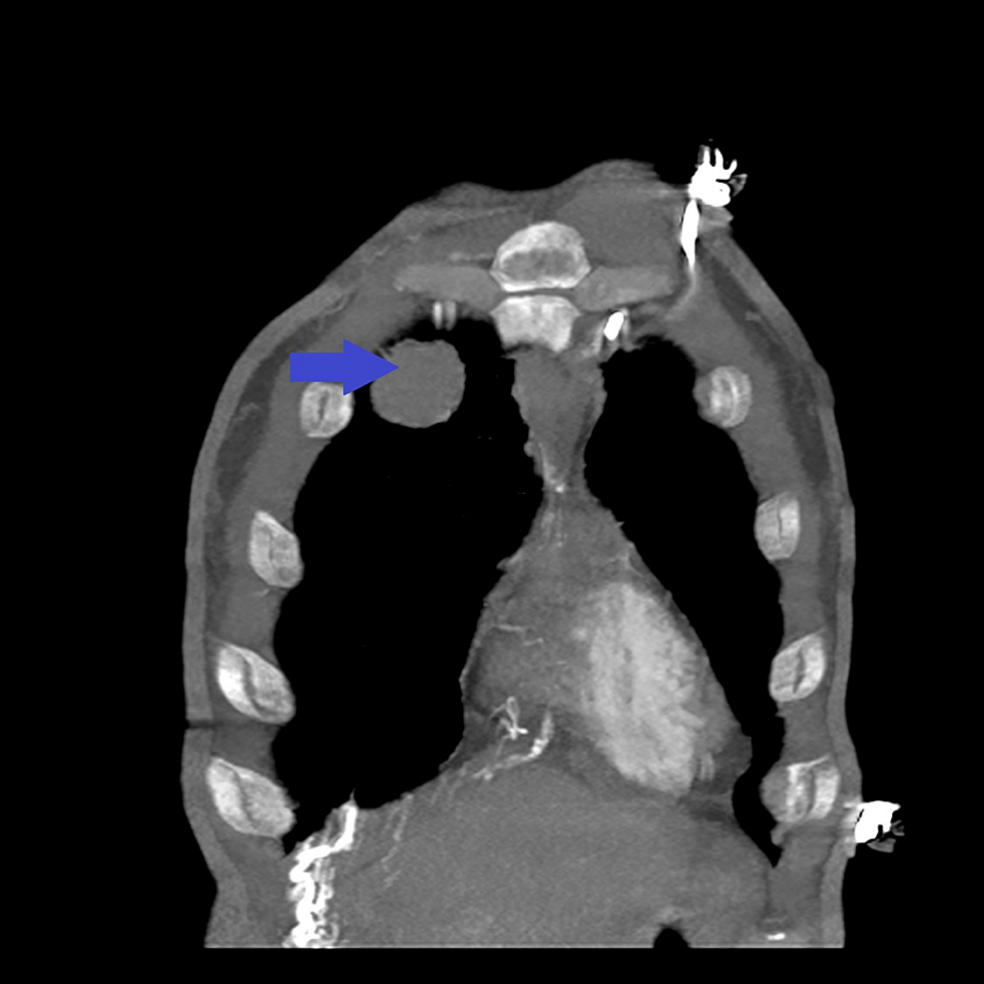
Enhanced CT scan of the chest showing a large metastasis to the right upper lobe (blue arrow).
